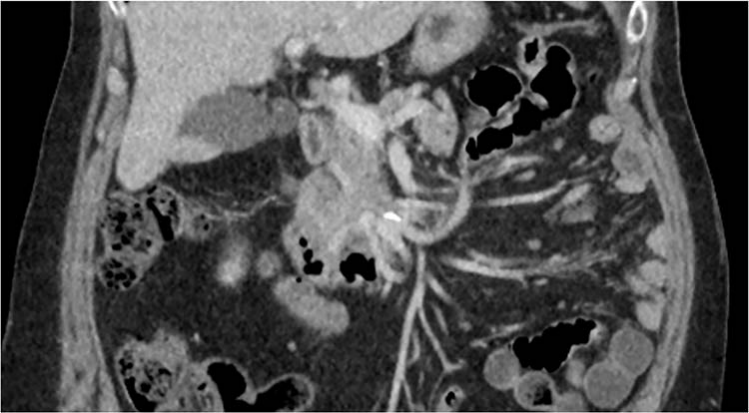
Initial CT: coronal view of a 13 mm hyperattenuating region with peripheral calcification at the inferior margin of the pancreatic uncinate process and the superior margin of the duodenum.
